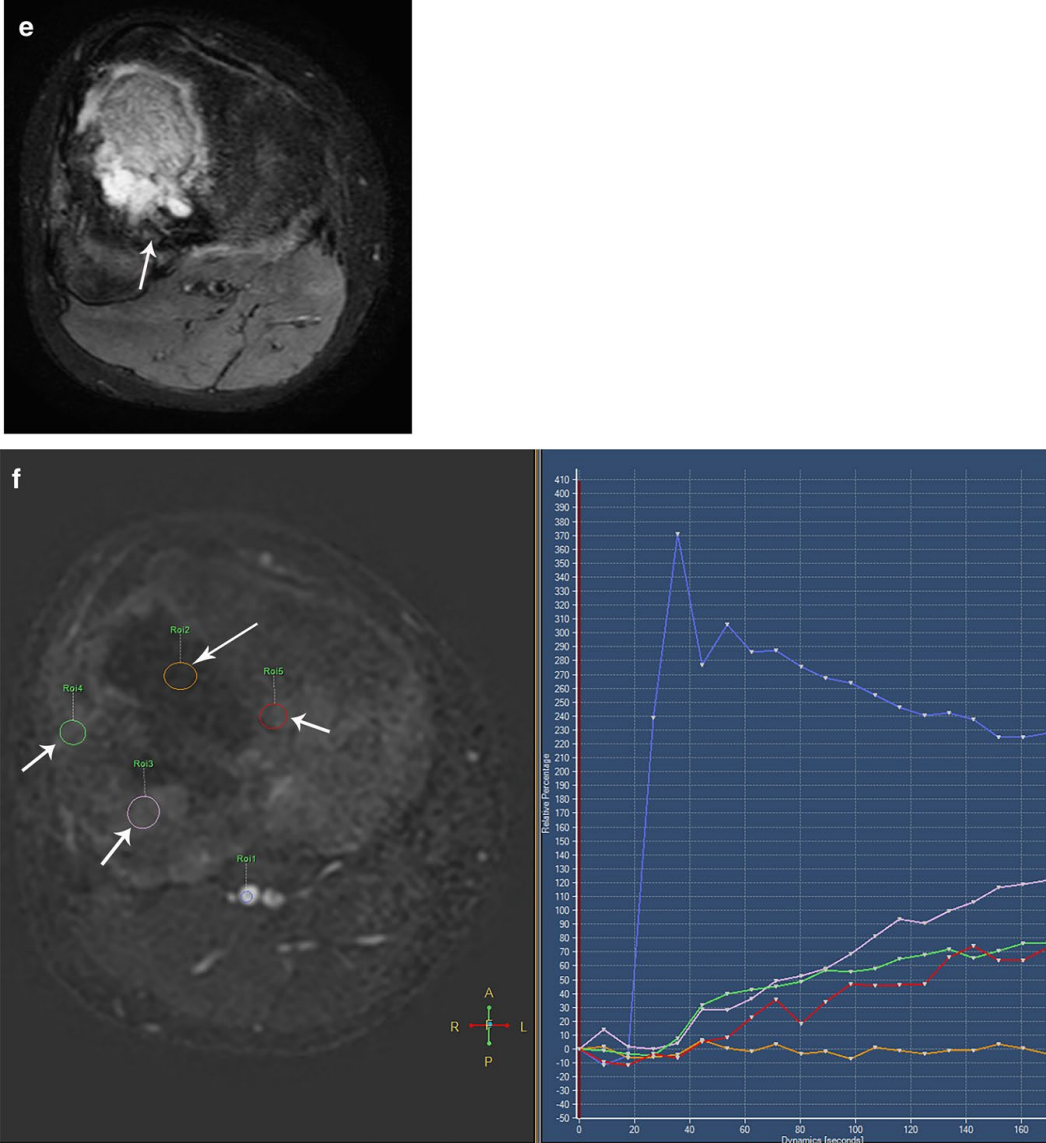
Osteosarcoma of the proximal right tibia in a 16-year-old boy. a Axial ADC map shows low signal intensity of the tumor confirming restricted diffusion (*). b Axial DCE-MR image (left) with corresponding time intensity curve (TIC) (right) shows peripheral enhancement of the tumor (short arrows on the left) which correlates with a type III curve (pink and orange arrows on the TIC). The blue curve in the TIC shows the typical morphology of any peripheral artery (long arrow on the left). c Axial fat-suppressed T2-weighted MR image shows hyperintensity of the bone marrow (arrows) surrounding the tumor (T), in keeping with edema. d Axial DCE-MR image (left) and corresponding TIC (right) show progressive enhancement of the bone marrow (arrows) which correlates with a type II curve (orange and pink curves), indicating edema. The blue curve in the TIC shows the typical morphology of the peripheral artery. e Axial fat-suppressed T2-weighted MR image after 2 cycles of chemotherapy shows an area of predominantly low signal intensity within the tumor (arrow) that may represent fibrosis and/or calcification. f Axial DCE-MR image (left) with corresponding TIC (right) shows progressive enhancement of the peripheral area of the tumor (short arrows) with a type II curve (red, green and pink curves), indicating overall good treatment response and presence of granulation tissue. Note the lack of enhancement of the central part of the tumor (long arrow) due to necrosis that correlates with a type I curve (orange curve) indicating non-viable tumor or necrosis. The blue curve correlates with the typical morphology of a peripheral artery
